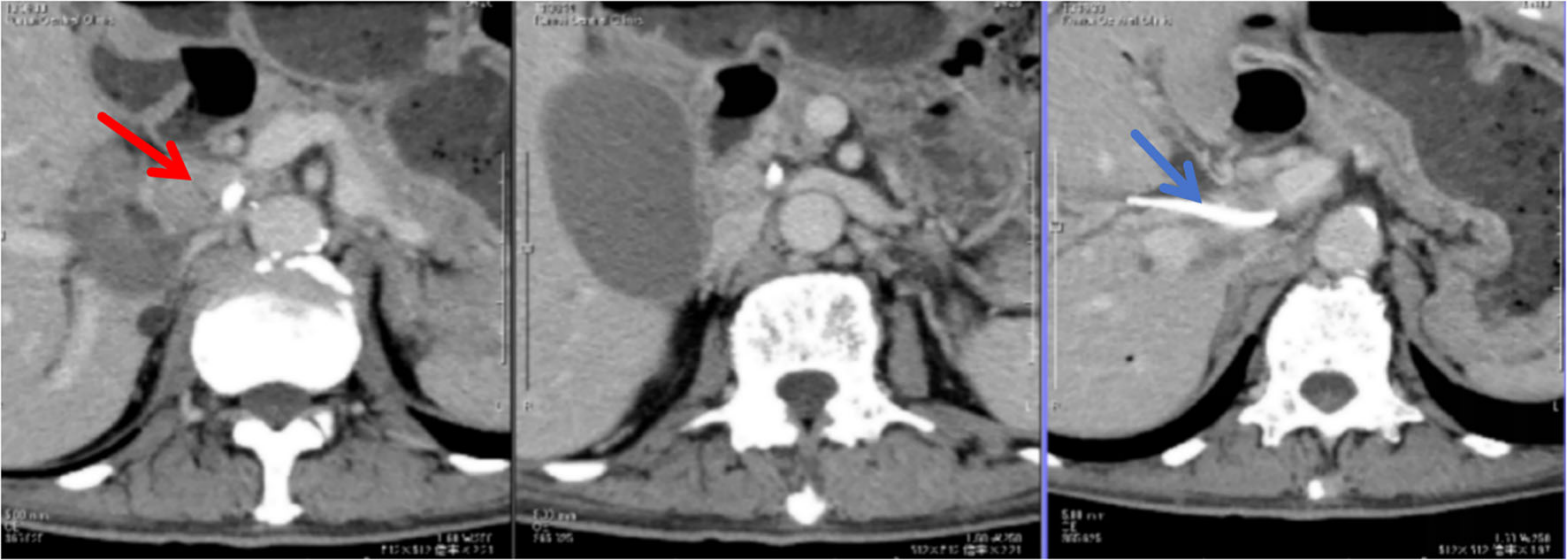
An abdominal computed tomography (CT) scan obtained following neoadjuvant chemotherapy, revealing a tumor located at the hepatic portal (indicated by a red arrow) and an indwelling biliary stent (indicated by a blue arrow).
